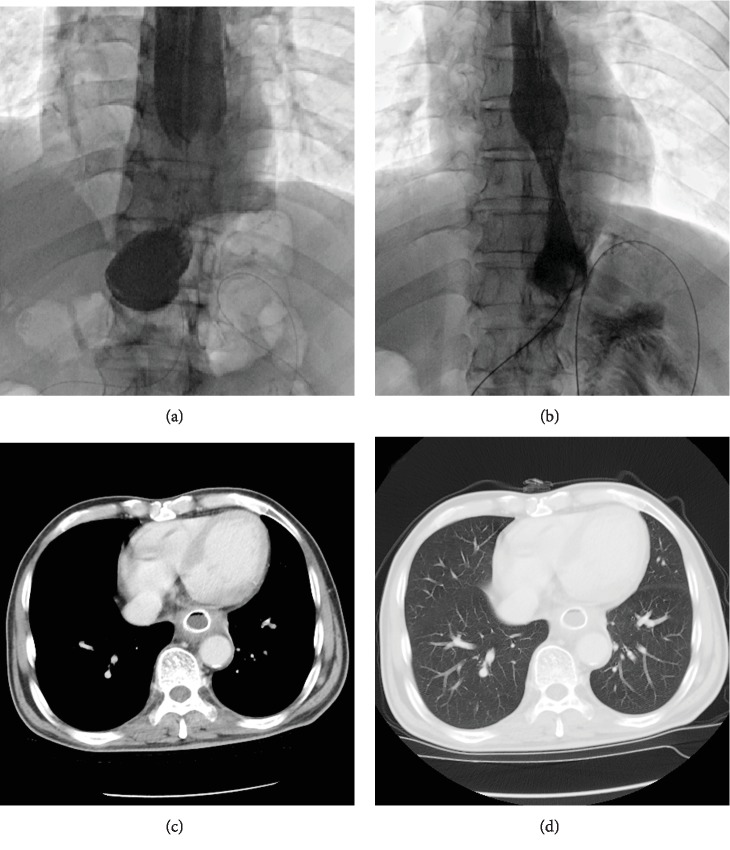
A 63-year-old man with anastomotic stenosis treated by stent placement (case 12). (a) Esophagography showed the site of anastomotic stenosis in the lower esophagus. (b) A covered stent was inserted. (c, d) At 48 days after stent placement, a chest CT scan shows well expansion of stent and relief of stenosis.
